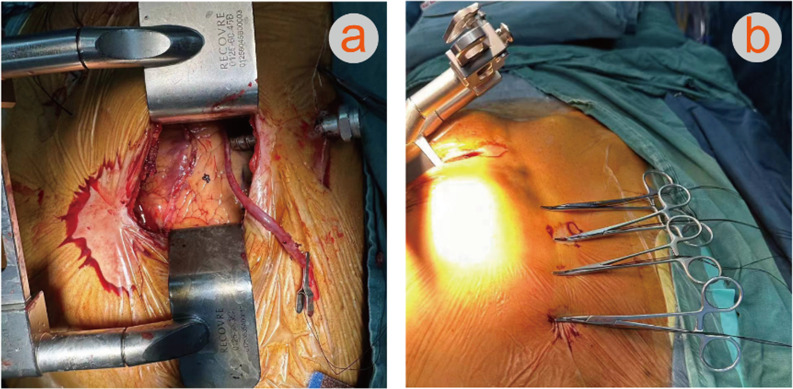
The site of main incision and skin puncture points for right pericardium suspension. (a) A 5–8 cm skin incision in the left fifth intercostal thoracotomy following ThoraTrak retractor position. (b) right pericardium suspension using stitches through the second to sixth intercostal parasternal spaces
